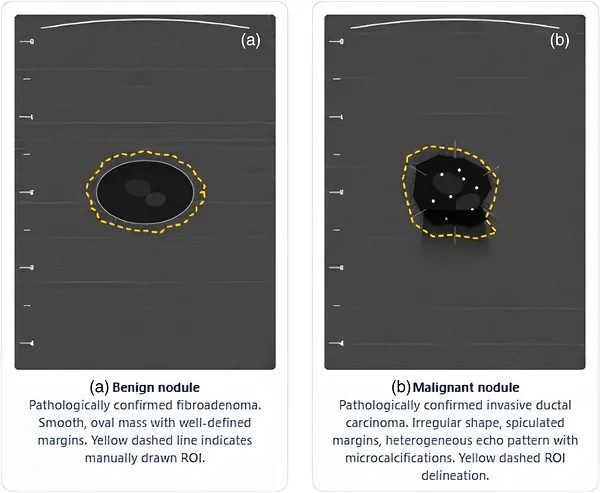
Representative grayscale ultrasound images with ROI delineation for BI‐RADS 4a nodules. (a) Benign nodule (pathologically confirmed fibroadenoma) with manually drawn ROI overlay; (b) Malignant nodule (pathologically confirmed invasive ductal carcinoma) with manually drawn ROI overlay.
